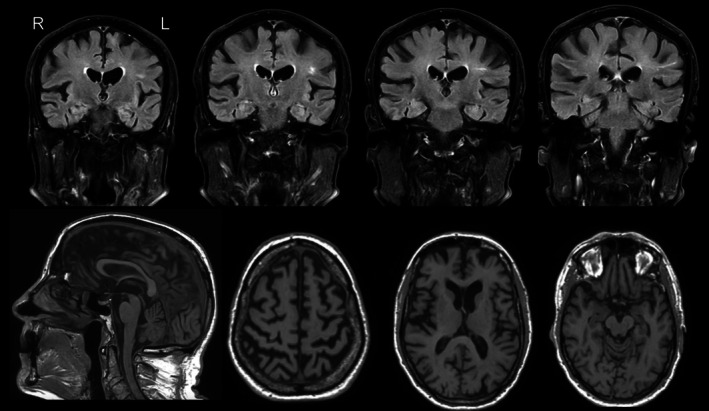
Representative MRI images taken when the patient was aged 81 years. MRI revealed mild asymmetric left greater than right frontotemporal atrophy and some linear white matter hyperintensity in the left frontal area.
